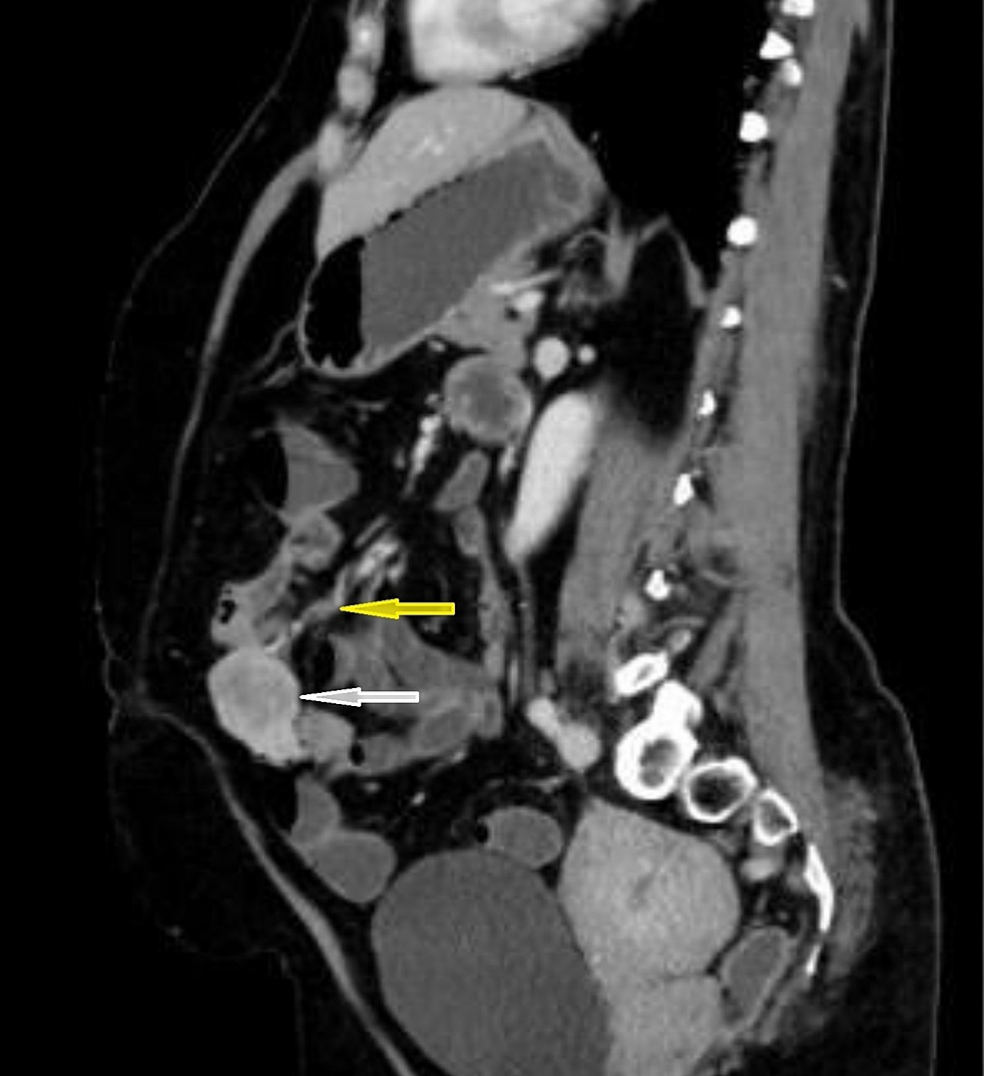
CT scan angiogram image showing the soft tissue lesion (the white arrow) with the feeding blood vessels from the central mesenteric blood vessels (the yellow arrow).
